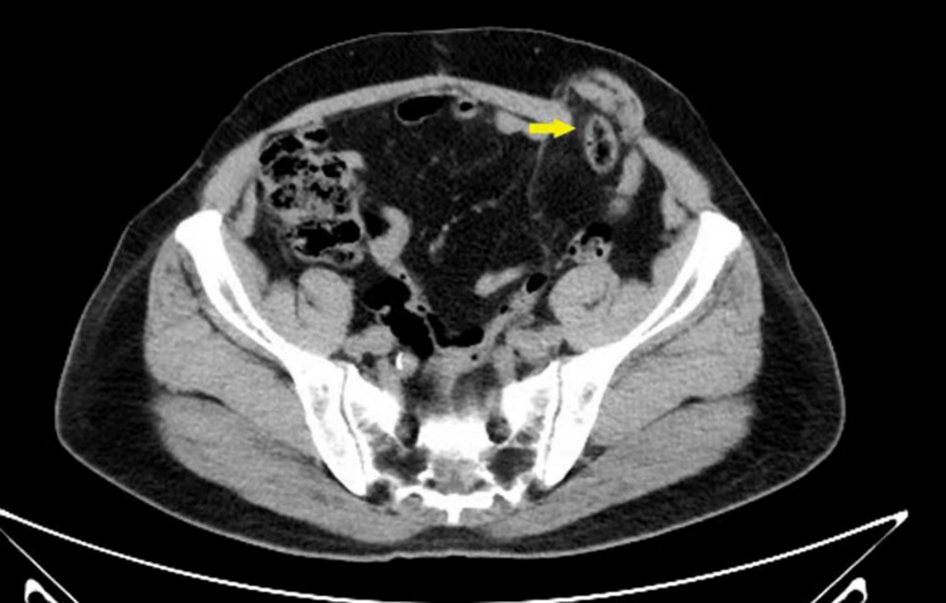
Pre-operative CT scan showing parastomal hernia (yellow arrow).
